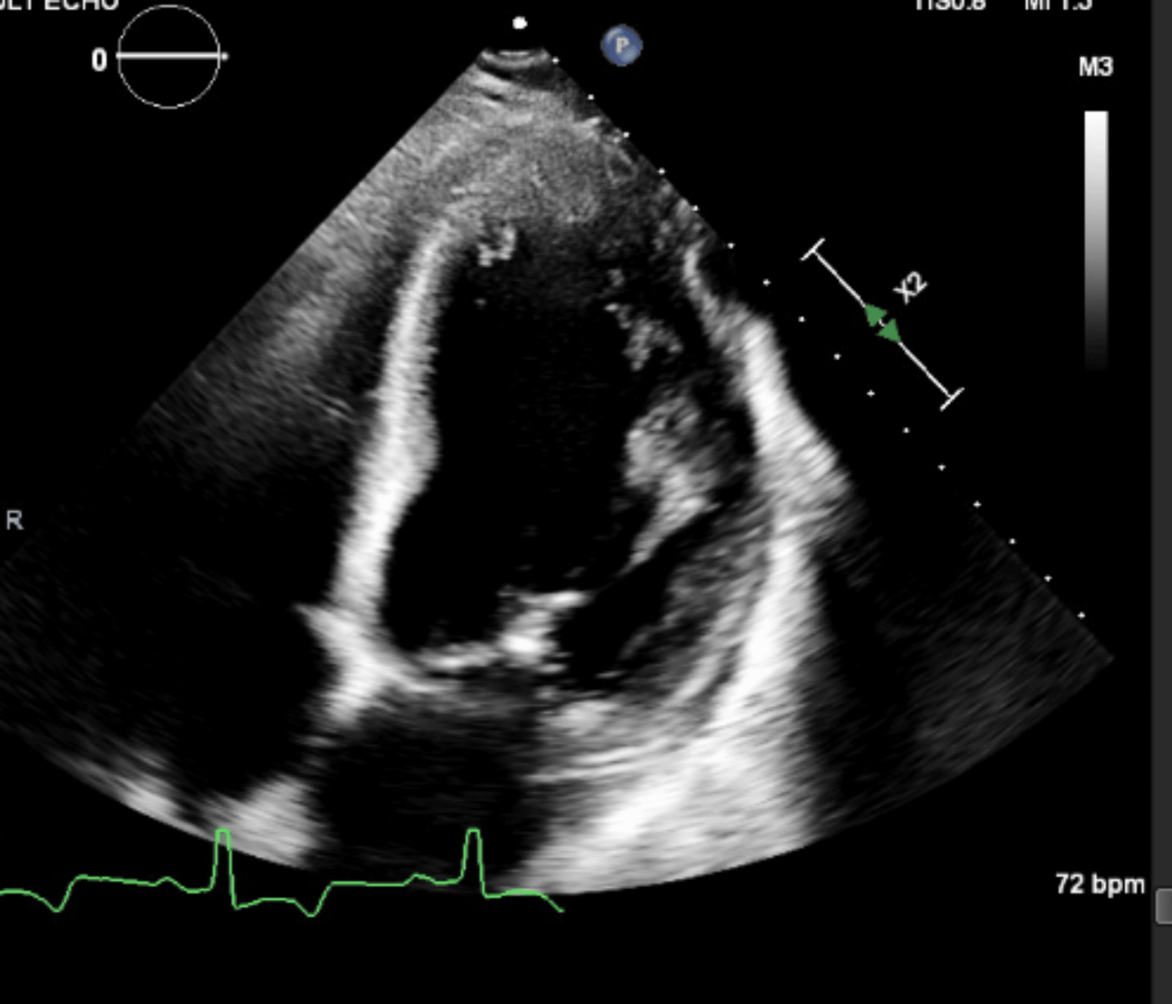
Transthoracic echocardiography images showing left atrial and ventricular dilatation and hypokinesis, indicating severe cardiac remodeling consistent with chronic rheumatic heart disease
